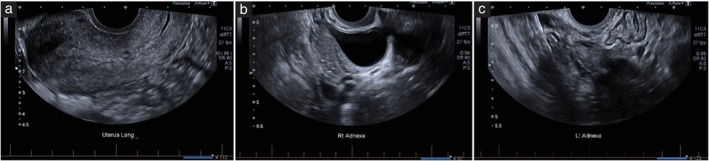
Example of one case (three ultrasound images) reviewed by raters. For each case, ultrasound images in the midsagittal plane of the uterus (a), right ovary (b) and left ovary (c) were reviewed. All images reviewed were single‐screen images, free of measurement calipers and color Doppler boxes. Anatomical annotations remained on images; however, information that could identify the patient or clinic from which the image was acquired was removed before starting the study. Lt, left; Rt, right.
